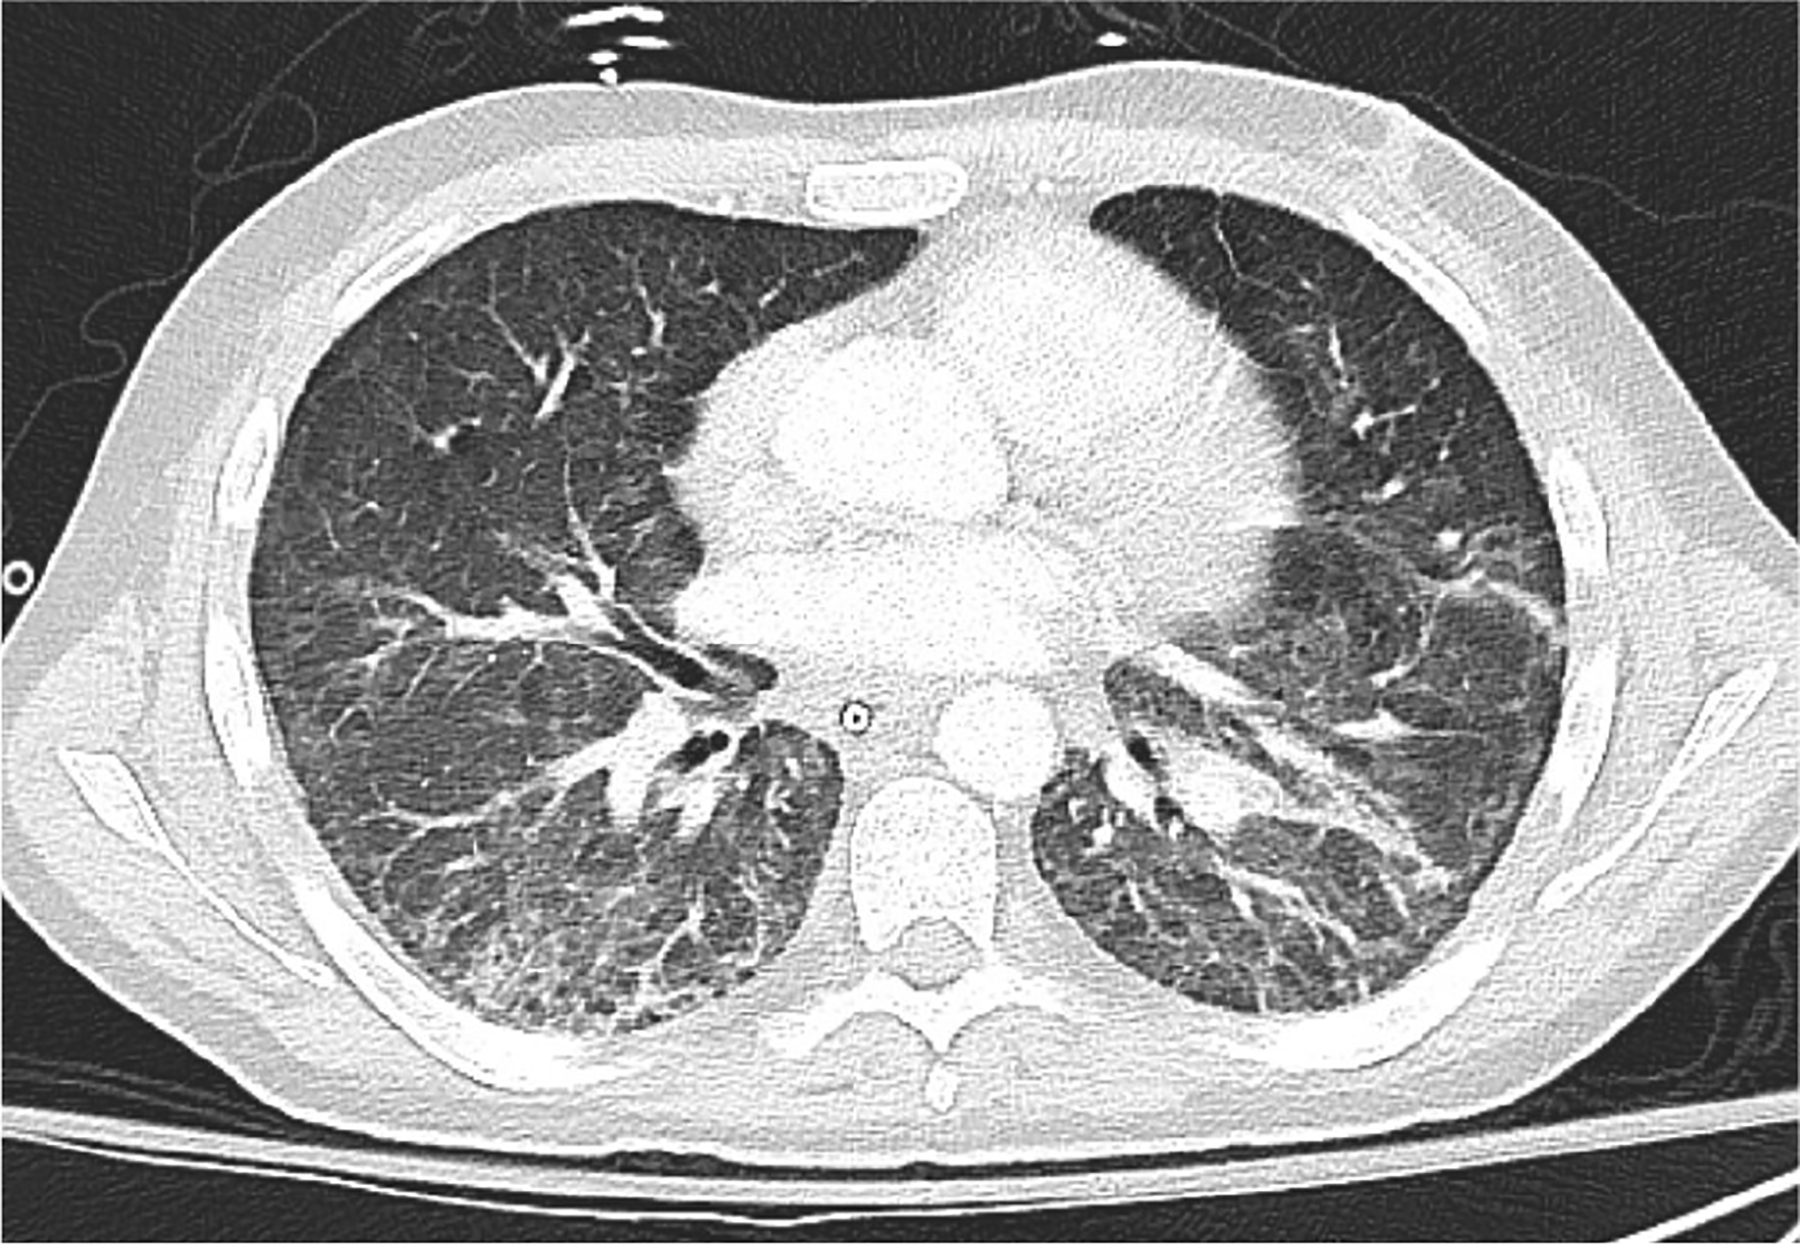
Axial CT image, corresponding with hospital day 49 in Figure 1. Compared to Figure 2, there is significant improvement of the patchy bilateral airspace consolidation. There remains diffuse, centrilobular ground glass opacities on a background of interlobular septal thickening with minimal consolidative changes in the lung bases. There are no new areas of consolidation.
